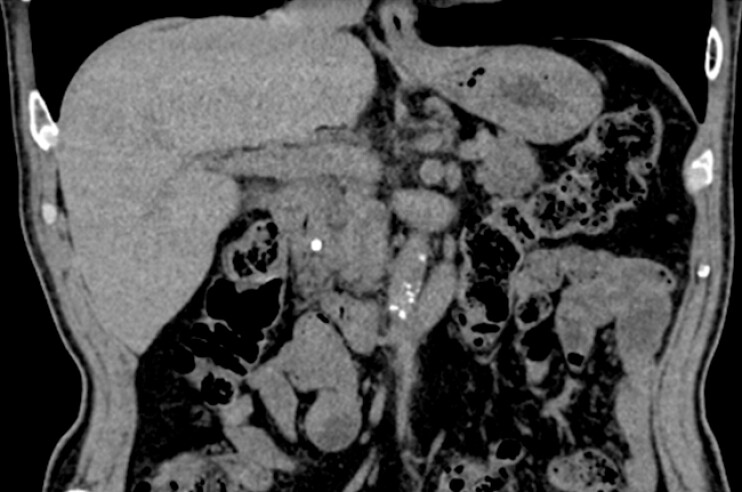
Abdominal computed tomography revealed a 5-mm stone in the duct of Santorini with associated pancreatic duct dilation.
